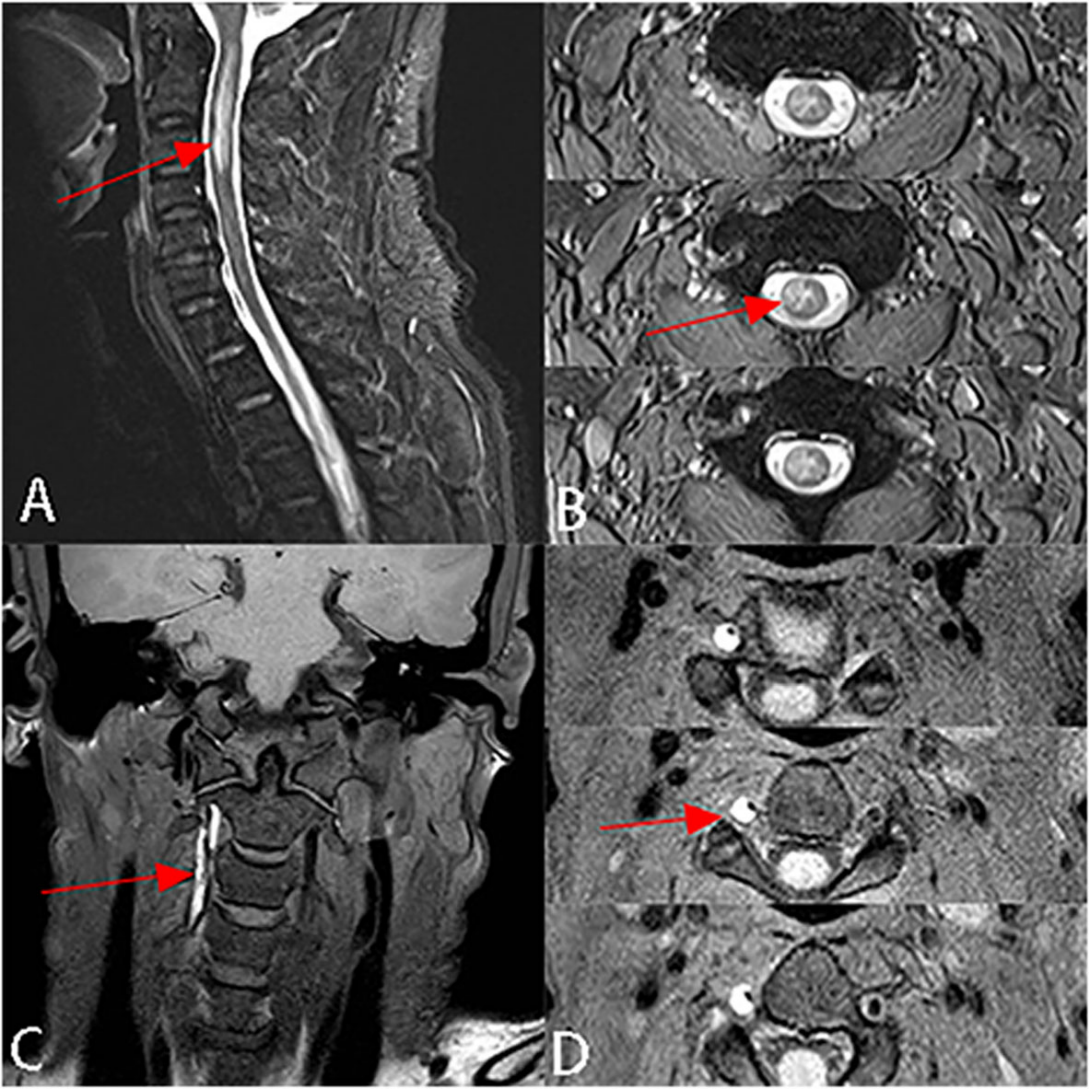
a Sagittal T2-weighted magnetic resonance image of cervical spine reveals spinal cord enlargement with hyperintense lesion at the level of C1–3 (red arrow). b Axial T2WI shows hyperintense signal of right spinal cord (red arrow). This is consistent with spinal cord infarction (SCI). (c)(d) HR-MRI VISTA sequence shows narrowing of the right vertebral artery at the level of C1–3 with eccentric high signal parallel to the narrowed lumen (red arrow)
